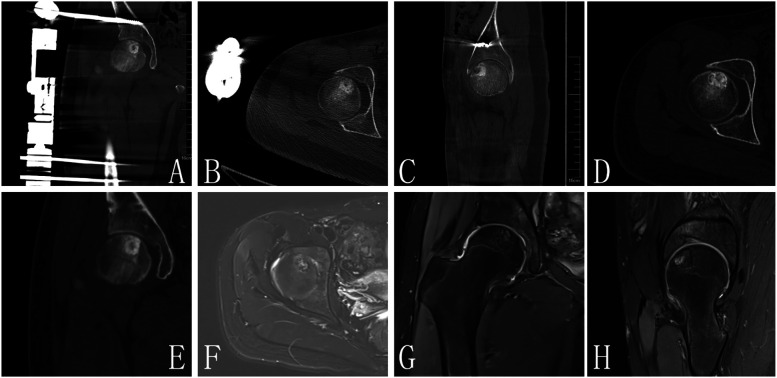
(A–C) Are postoperative images, CT (D,E) and MRI (F–H) of 1 year FU demonstrated no evidence of lesion recurrence in the femoral head, with radiological signs indicating complete resolution of the previous lesion.
